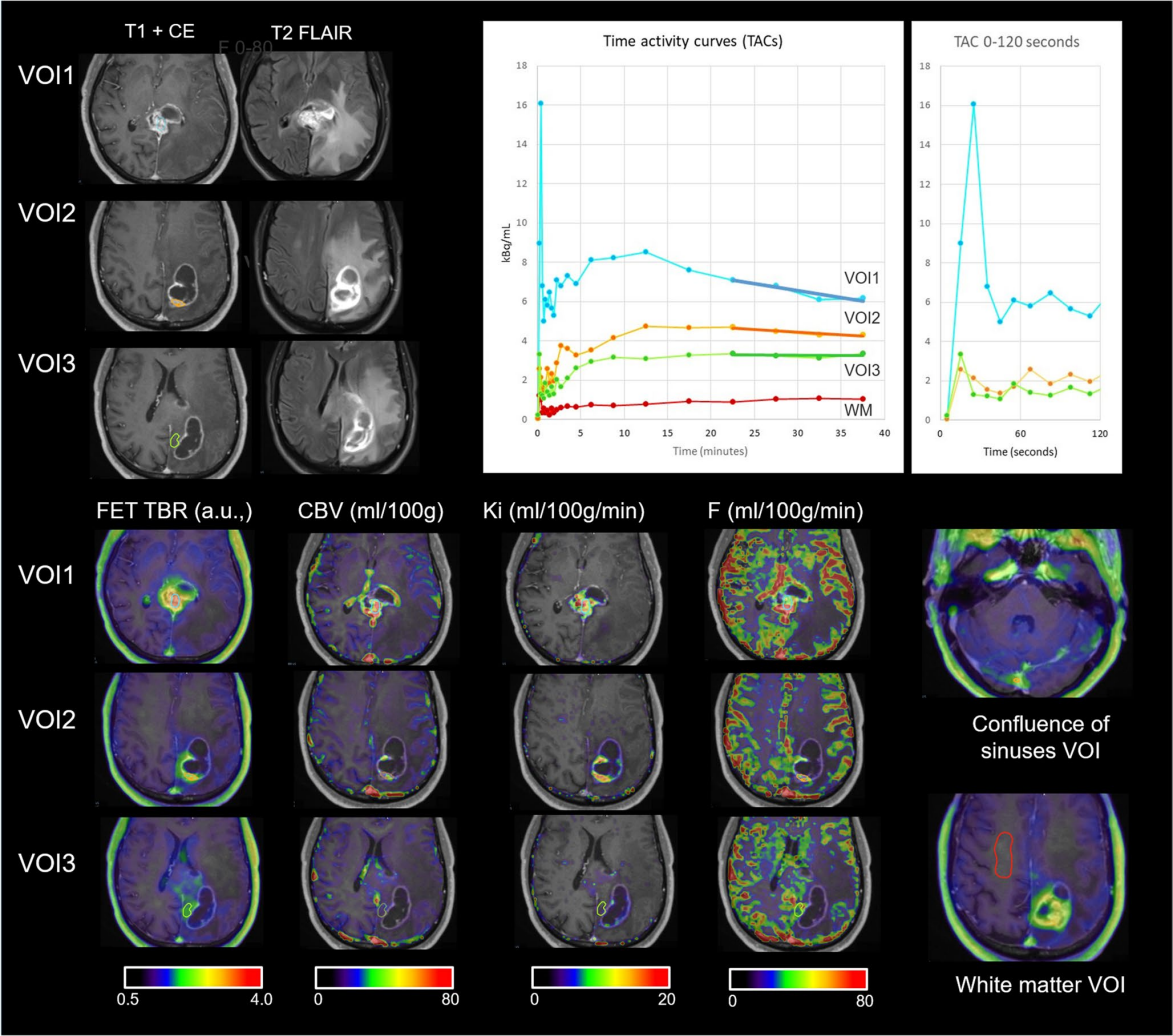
Example of VOI based analysis. Figure shows normal appearing white matter and lesion VOIs on MRI, static [^18^F]FET, DCE parameter maps and associated time activity curves (TACs) from a patient with multifocal recurrent glioblastoma. Linear fit of last part of the TAC used for calculation of normalized slope is shown, and first 120 s TAC for calculation of iAUC_120_ is presented in separate plot. Three lesion sub-VOIs representing high (VOI1, light blue) and moderately (VOI2, amber) active contrast enhancing, and non-enhancing (VOI3, light green) sub-VOIs
